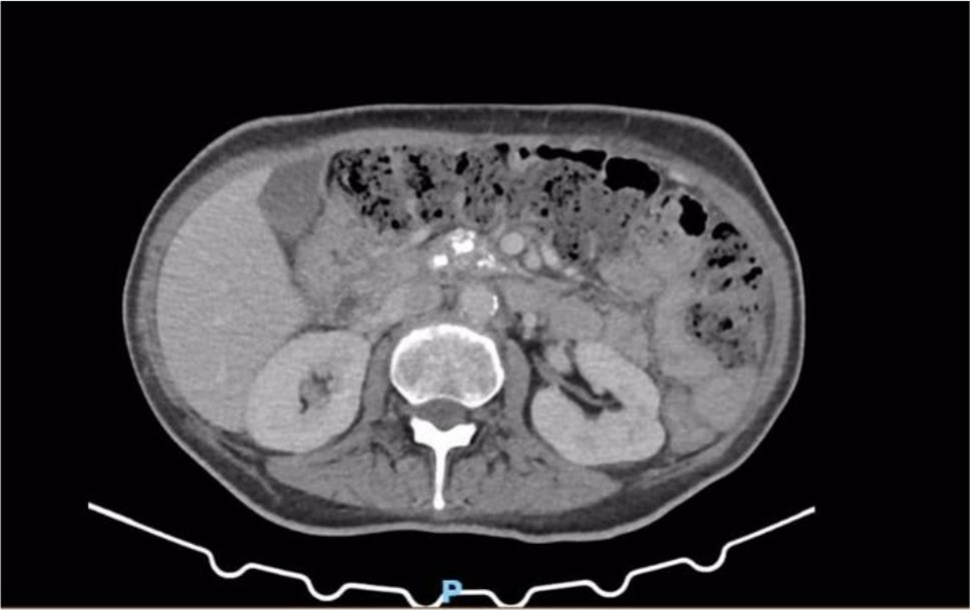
Abdominal CT scan before onset of symptoms showing no alterations of the gallbladder wall
